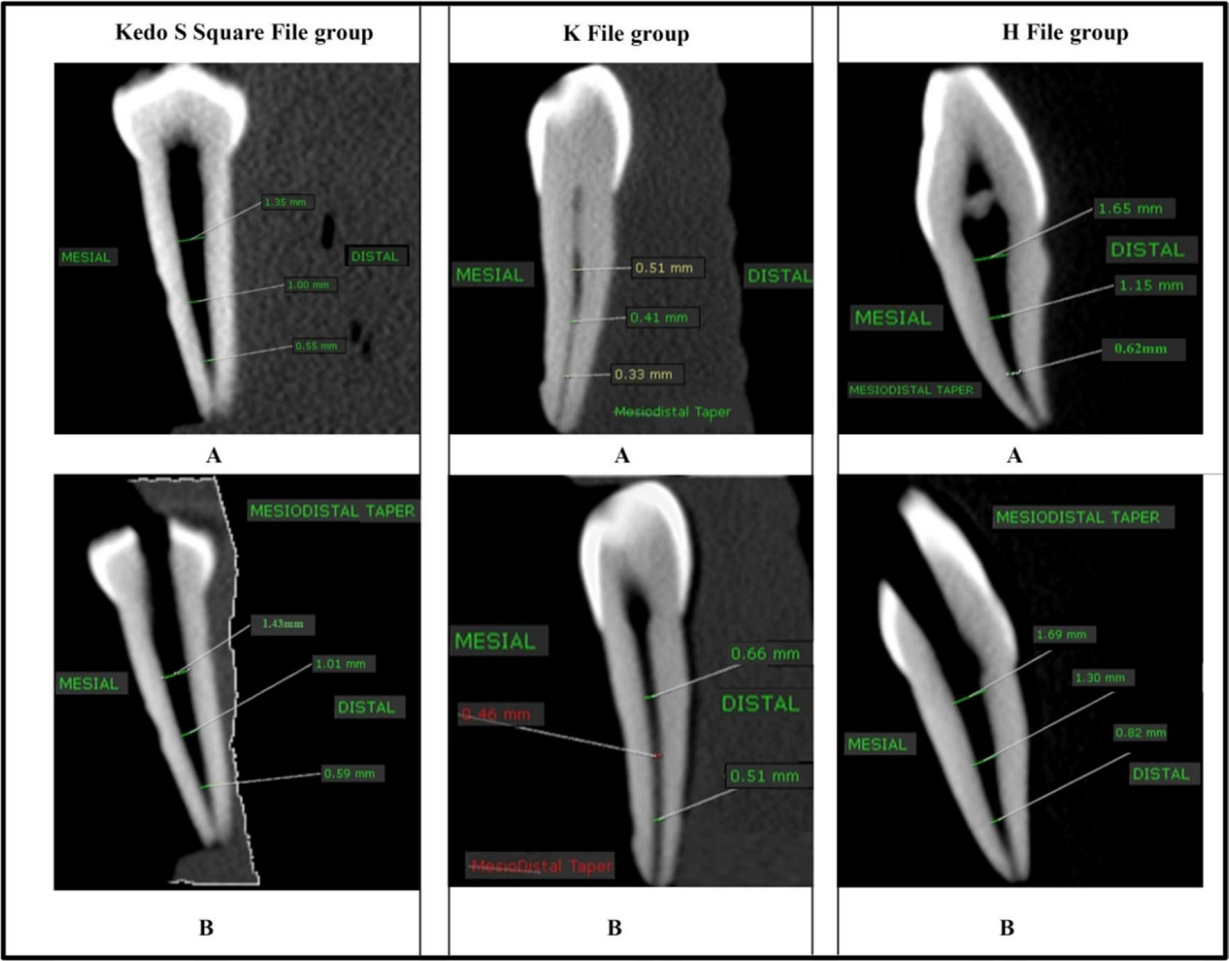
Pictorial representation showing sagittal cut of CBCT: a Pre- and Post-preparation CBCT scan images showing good taper with Kedo-S Square rotary file. b Pre- and Post-preparation CBCT scan images showing poor taper with manual K and H files
